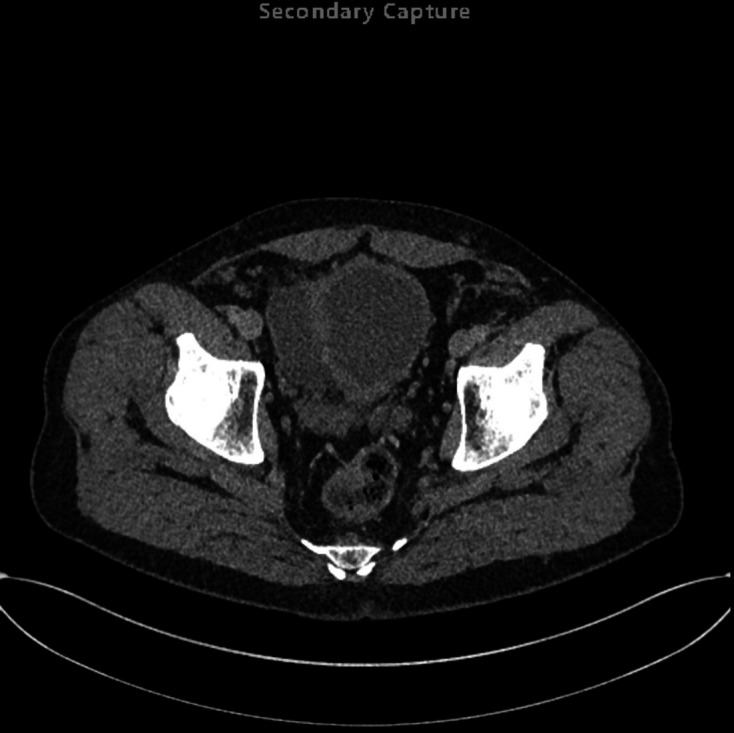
Third axial delayed phase CT (3 months following admission), on soft tissue
